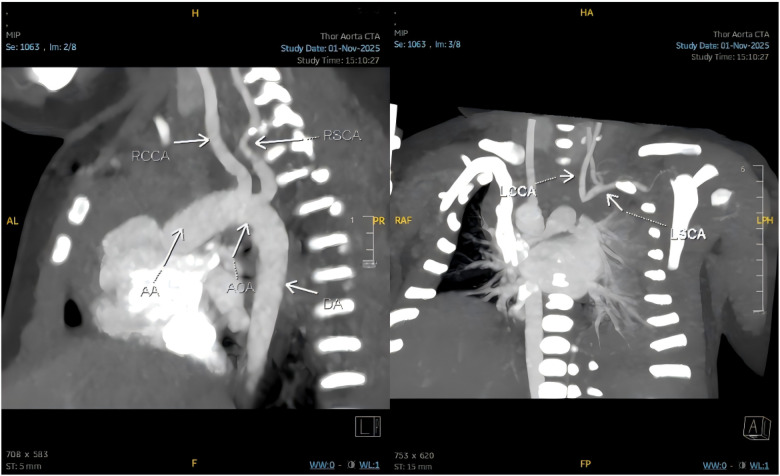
Chest computed tomography angiography confirming a right aortic arch with an isolated left innominate artery (RCCA, right common carotid artery; RSCA, right subclavian artery; AA, aortic arch; AOA, aortic arch origin; DA, descending aorta; LCCA, left common carotid artery; LSCA, left subclavian artery).
